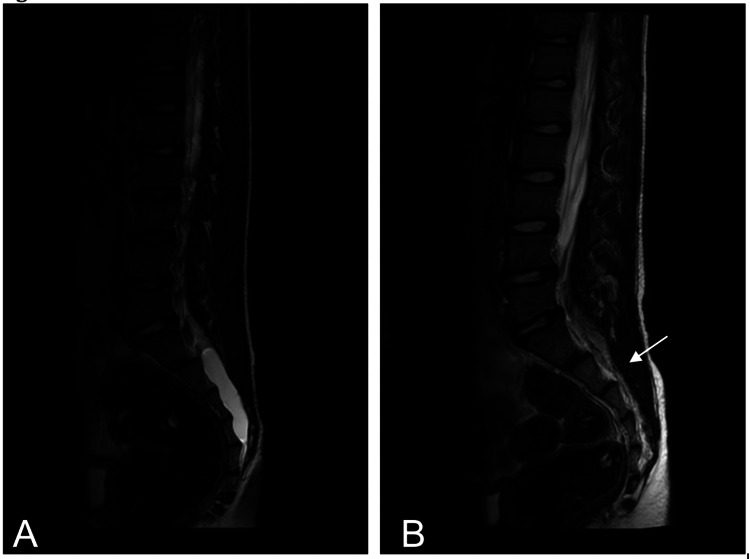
MRI T2 lumbar spine - sagittal view A represents the preoperative scan (five months prior to surgery) and B is the postoperative scan (four months after surgery) that reveals the complete resolution of the cyst and lack of recurrence. The arrow indicates the location of the tethered cord.
